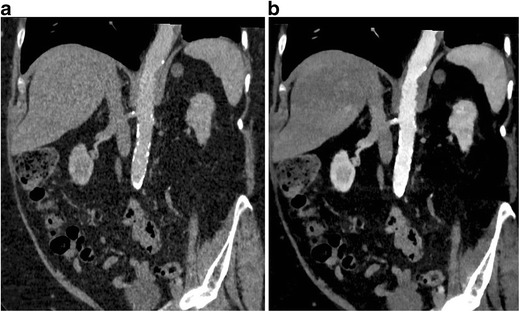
Low-contrast dose study. (a) Coronal 120-kVp routine diagnostic CTA image in a patient who received only 20 ml of intravenous contrast, shows poor contrast opacification of the abdominal aorta as well as parenchymal organs. (b) 40 keV VMI at the same level shows significant improvement of vascular contrast in the abdominal aorta. Thus, the low-energy VMI allows the use of low contrast dose, which is valuable in patients with severe renal dysfunction
